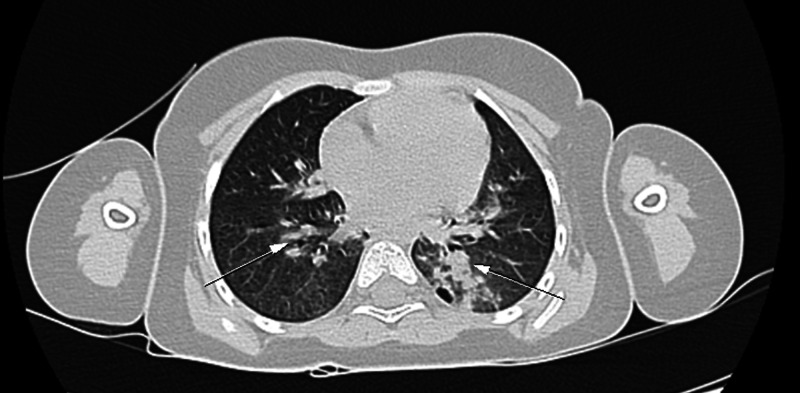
Chest CT scan showing bilateral parenchymal condensation (arrows)
